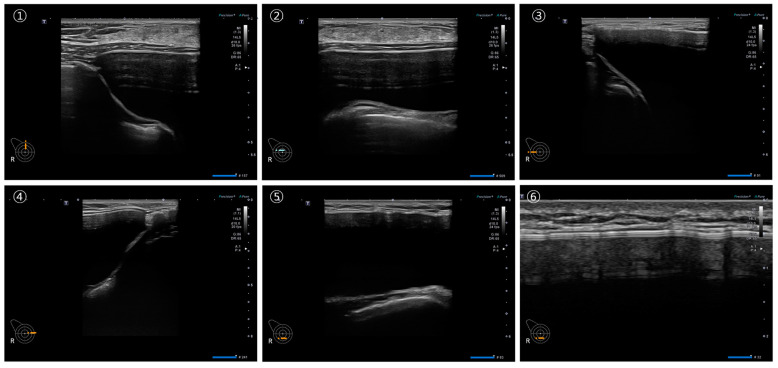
Examples of breast implant ultrasonography. ① US image of the lateral part of the breast implant and periprosthetic space, ② US image of the central part of the breast implant and the periprosthetic space, ③ US image of the medial part of the breast implant and the periprosthetic space, ④ US image of the upper part of the breast implant and the periprosthetic space, ⑤ US image of the lower part of the breast implant and the periprosthetic space, and ⑥ US image of the shell surface topography of the breast implant.
